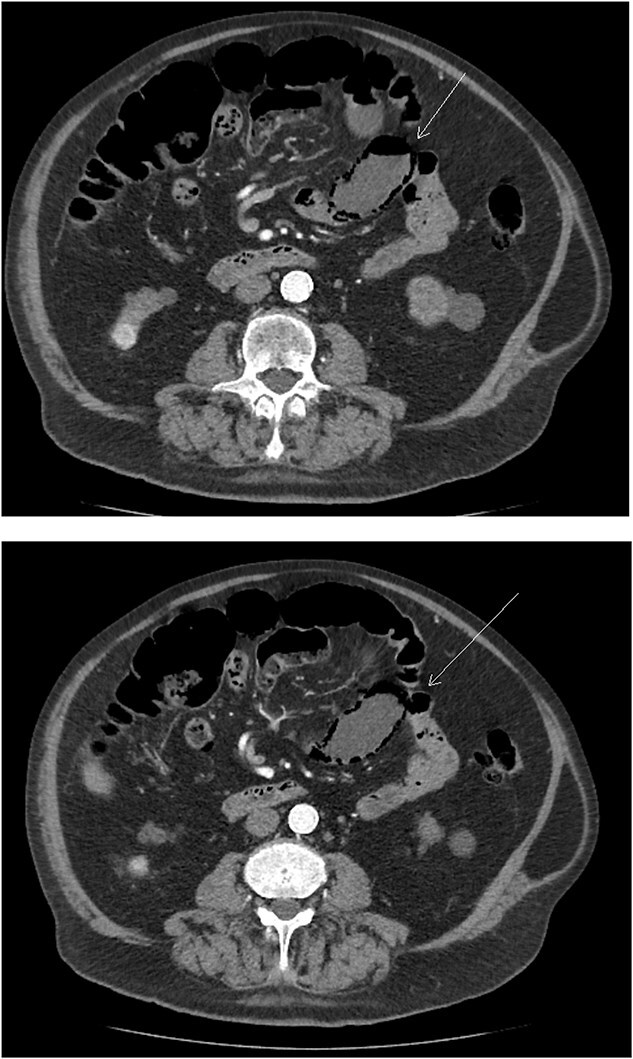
Axial abdominal CT scan image where the arrow points to the presence of pneumatosis intestinalis.
